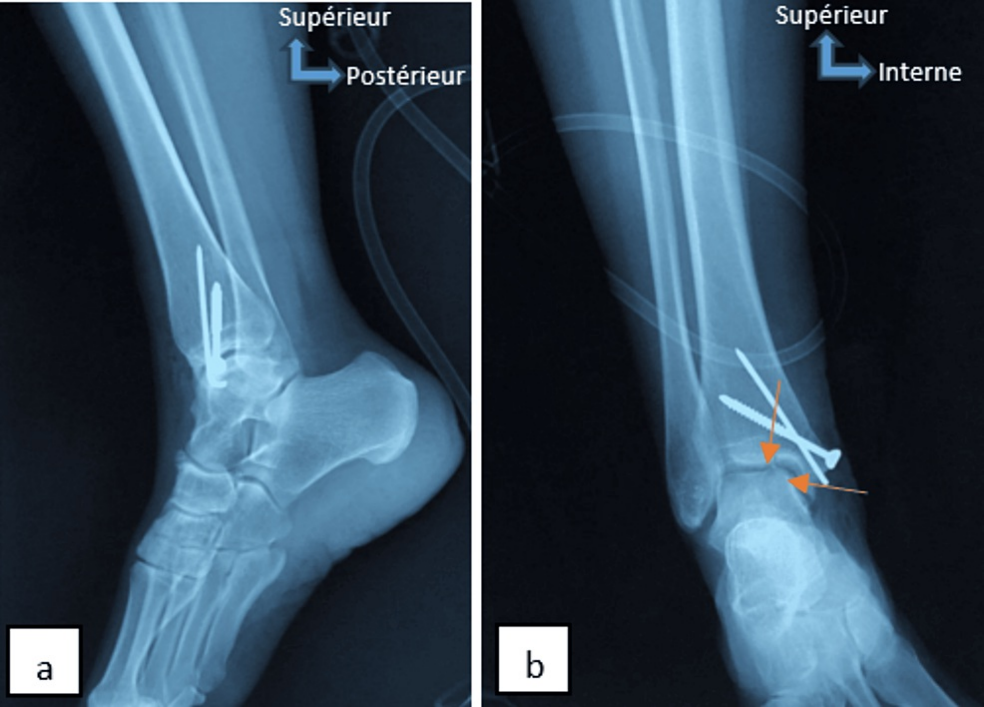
Postoperative control radiograph (face (a), profile (b)) shows medial malleolus fixation hardware and osteochondral graft in place (orange arrows).
